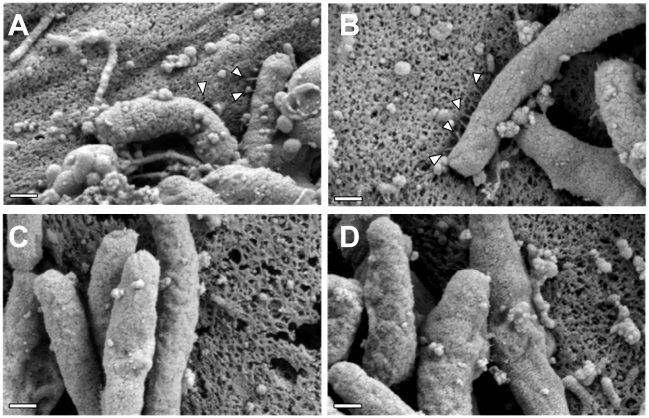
The C-terminal motif of CagI and CagL is required for pilus formation. WT *H. pylori* or mutant strains expressing C-terminally (CT) truncated forms of CagH, CagI, or CagL were co-cultured with AGS cells and imaged by FESEM. (A) WT. (B) CagH-HAΔCT. (C) CagI-FLAGΔCT. (D) CagLΔCT. Magnification bars indicate 200 nm.
